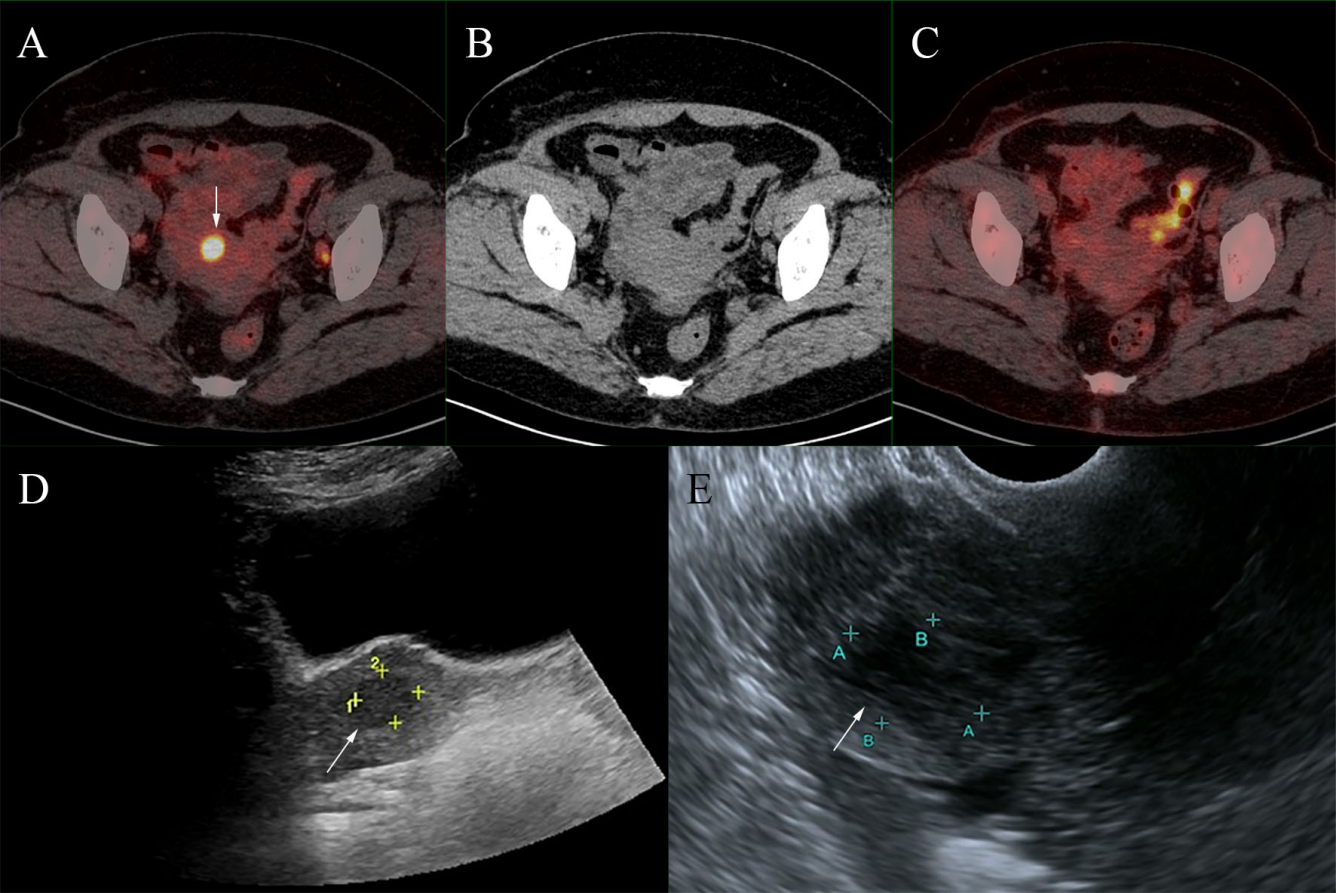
A foci of increased ^68^Ga-DOTATATE activity (A, fused PET/CT) was located in the uterus (SUVmax,11.0; white arrows), but neither notable CT structural alterations nor ^18^F-FDG activity was seen (B, CT; C, fused PET/CT). This lesion was found to be uterine fibroids after ultrasound examination (D, transabdominal ultrasonography; E, transvaginal ultrasonography).
